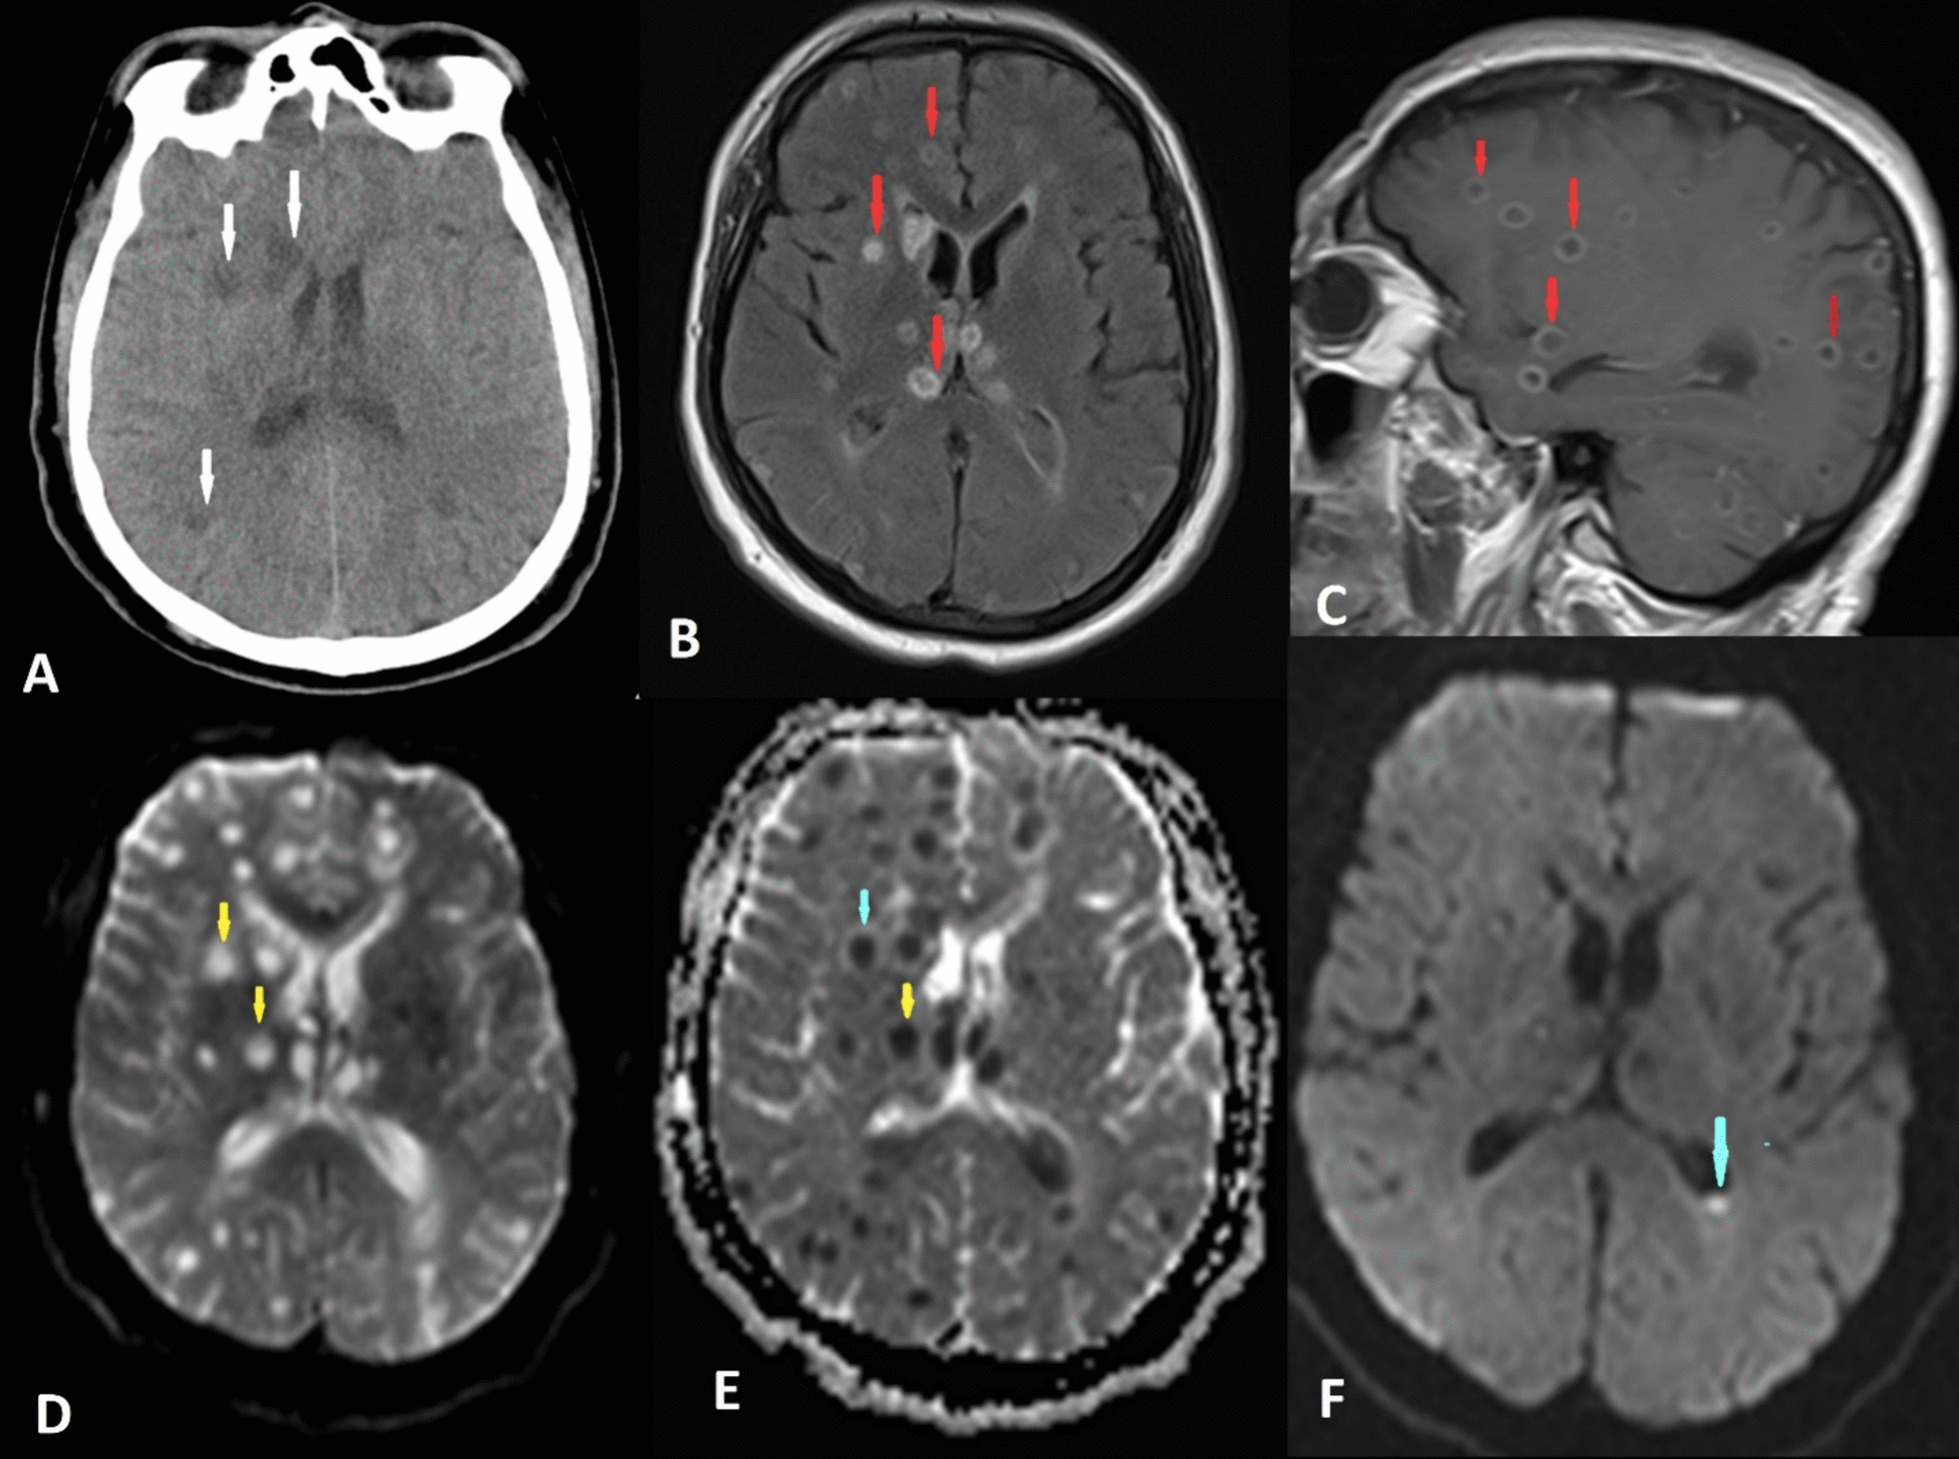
Brain computed tomography scan and magnetic resonance imaging at admission. A The brain computed tomography scan showed multiple supra- and infratentorial intra-axial hypodense lesions in both cerebral hemispheres and basal ganglia (white arrows). Axial FLAIR sequence, sagittal FLAIR sequence (B, C), axial T2W (D), and ADC map (E): Multiple distinct outlined intra-axial ring-enhancing lesions distributed within the supra- and infratentorial cerebral parenchyma, alongside the periventricular region (red and yellow arrows in B, C and D). Notably, the central portions of these lesions exhibit noticeable diffusion restriction, and there is mild peripheral edema surrounding the lesions (yellow and blue arrows in E). Brain MRI after treatment (F): Both the quantity and the extent of lesion restriction had visibly diminished. Furthermore, ventriculitis was confined to the left occipital horn (blue arrow in F)
